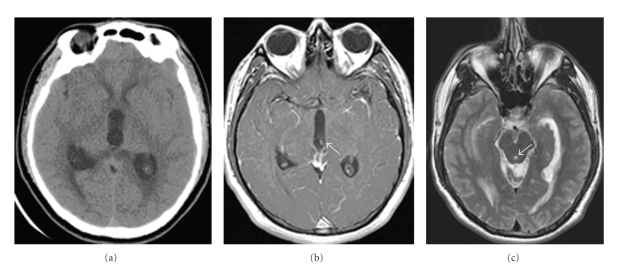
(a) CT head without contrast, (b) MRI T1 with contrast showing the cyst in the posterior third ventricle (arrow), and (c) MRI T2 showing altered flow through the Sylvian aqueduct (arrow).
